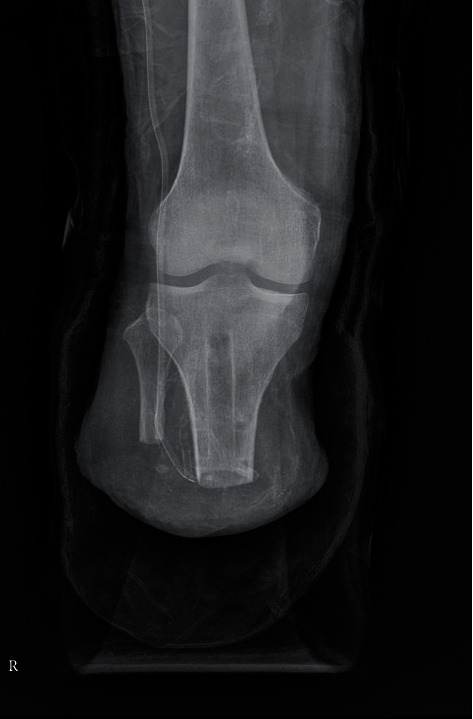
Postoperative plane radiograph. No hardware remained after surgery.
